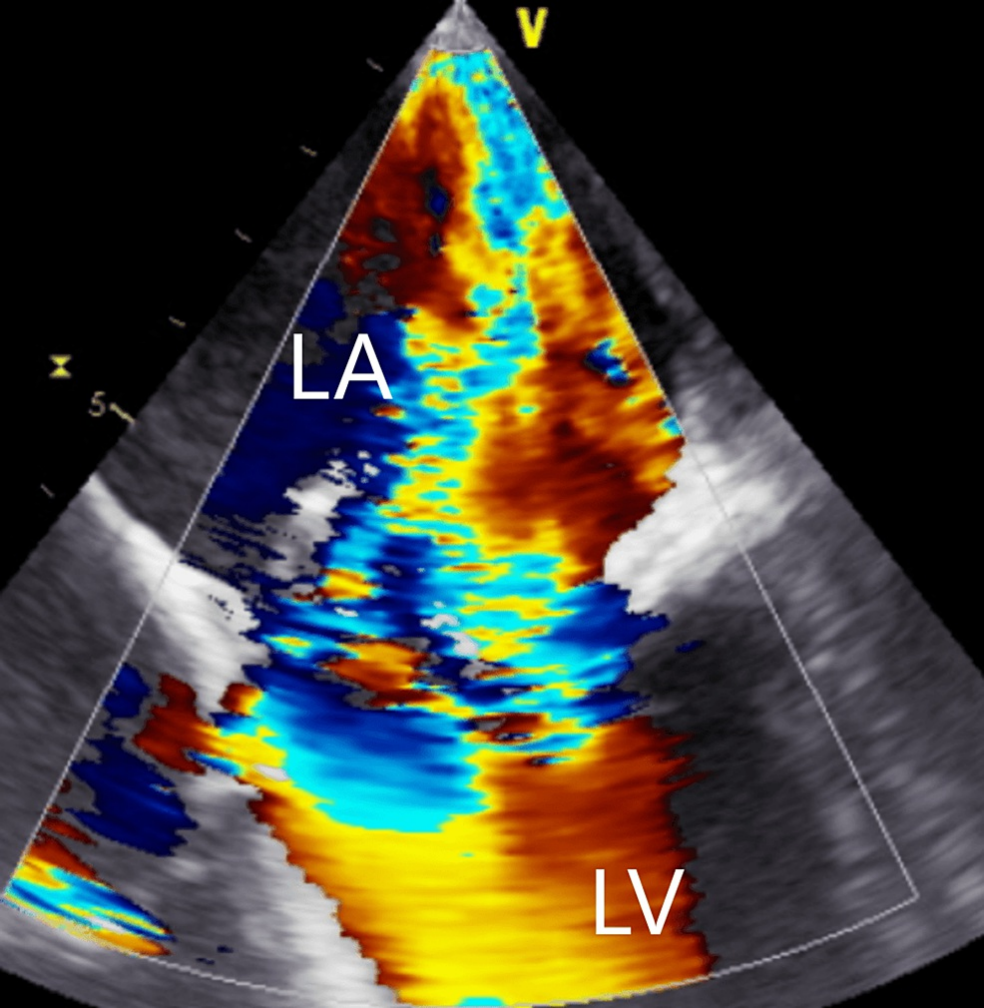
Intracardiac echocardiogram showing severe mitral regurgitation. LA, left atrium; LV, left ventricle
